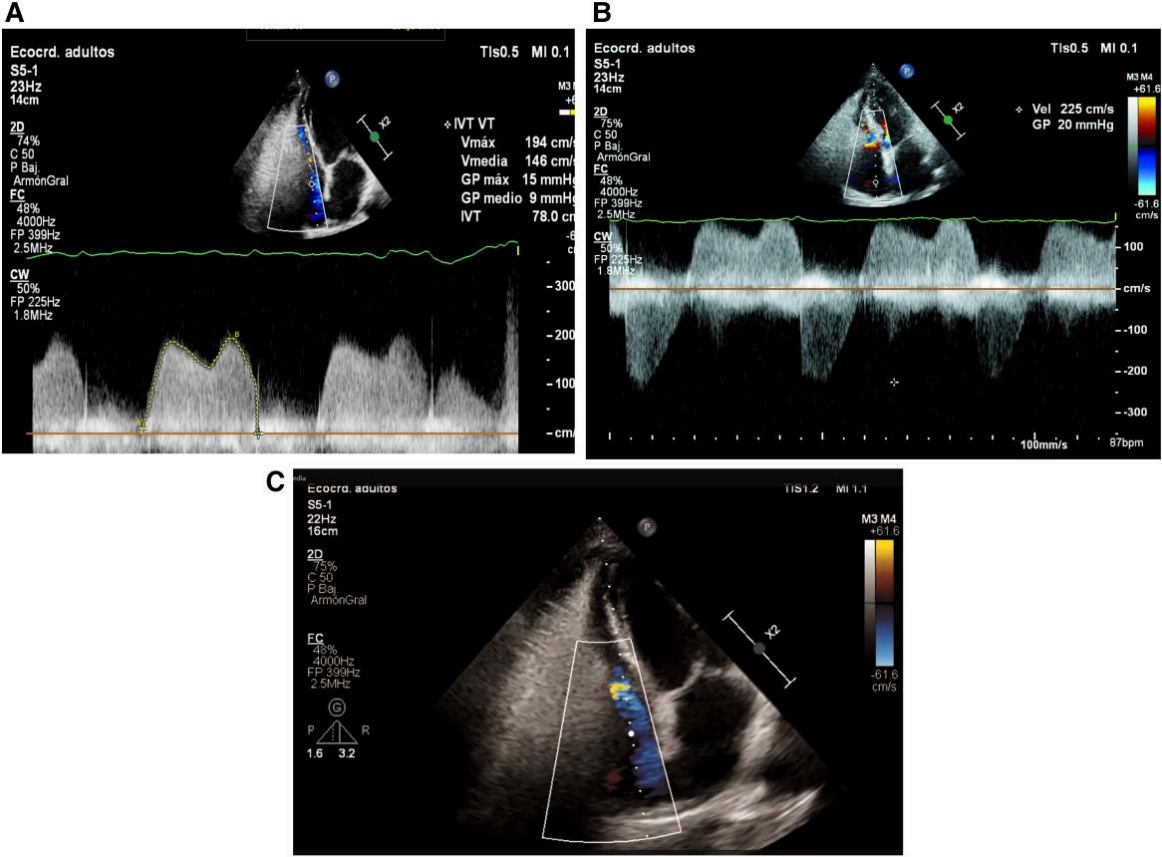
Mean gradient across the tricuspid valve (MG: 9 mmHg), consistent with severe tricuspid stenosis (*A*). Continuous-wave Doppler showing tricuspid regurgitation (*B*). Doppler image showing tricuspid regurgitation jet directed towards the interatrial septum (*C*).
